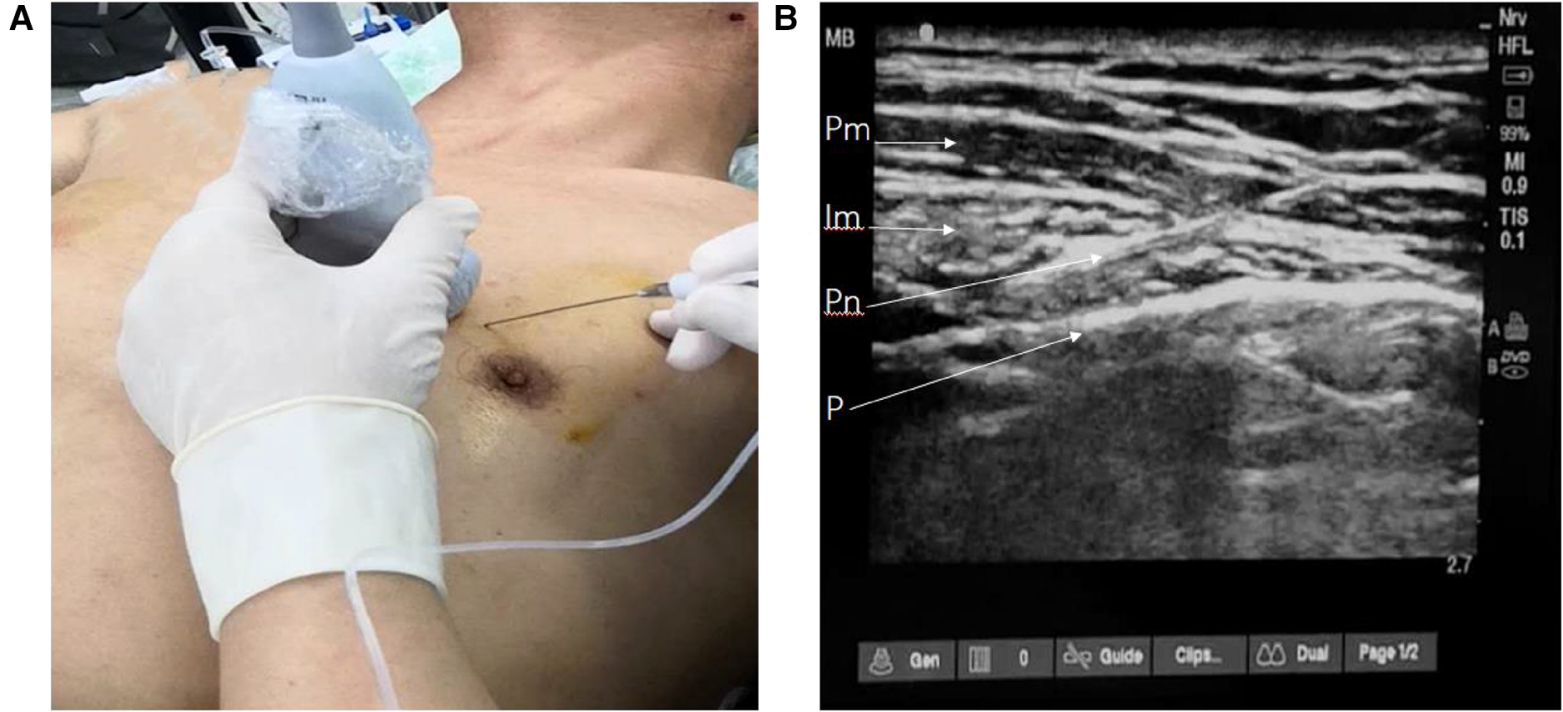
Parasternal intercostal nerve block procedure. (A) The needle and probe position, (B) ultrasonographic image of parasternal space. Pm, pectoralis major; Im,intercostal muscle; Pn, puncture needle; P, pleura.
